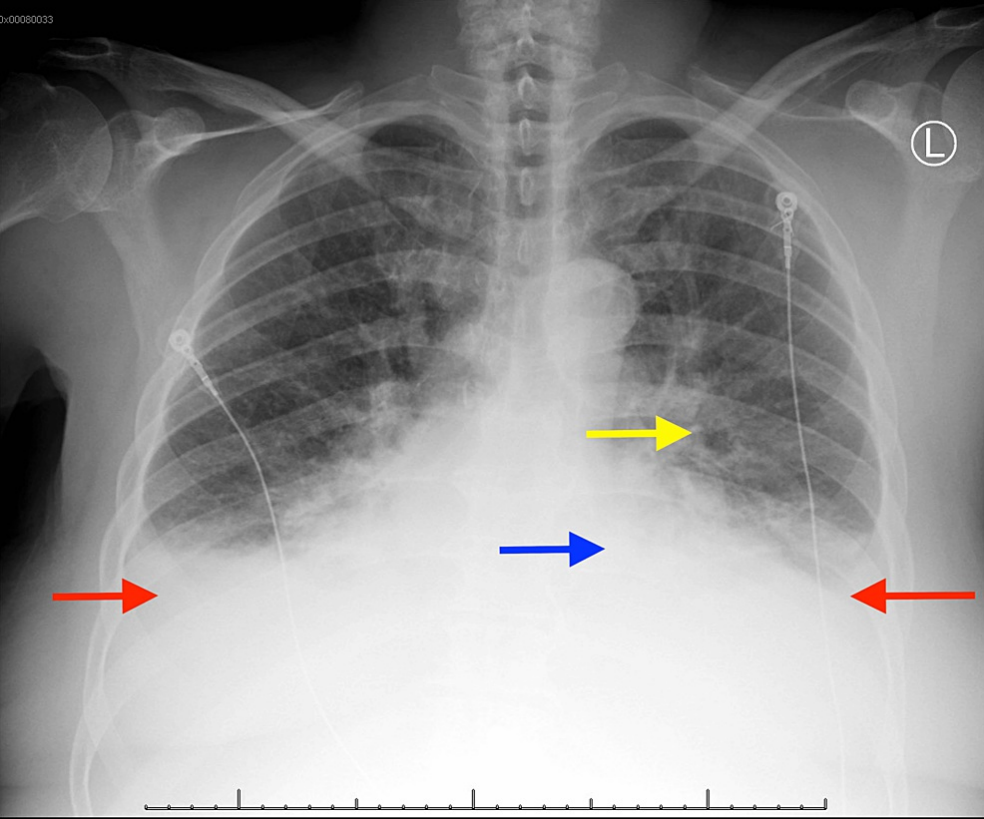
Chest x-ray showing bilateral pleural effusions and pulmonary vascular congestion suggestive of pulmonary edema Chest radiograph annotated demonstrating bilateral pleural effusions (red arrows), cardiomegaly (blue arrow), and pulmonary vascular congestion (yellow arrow)
